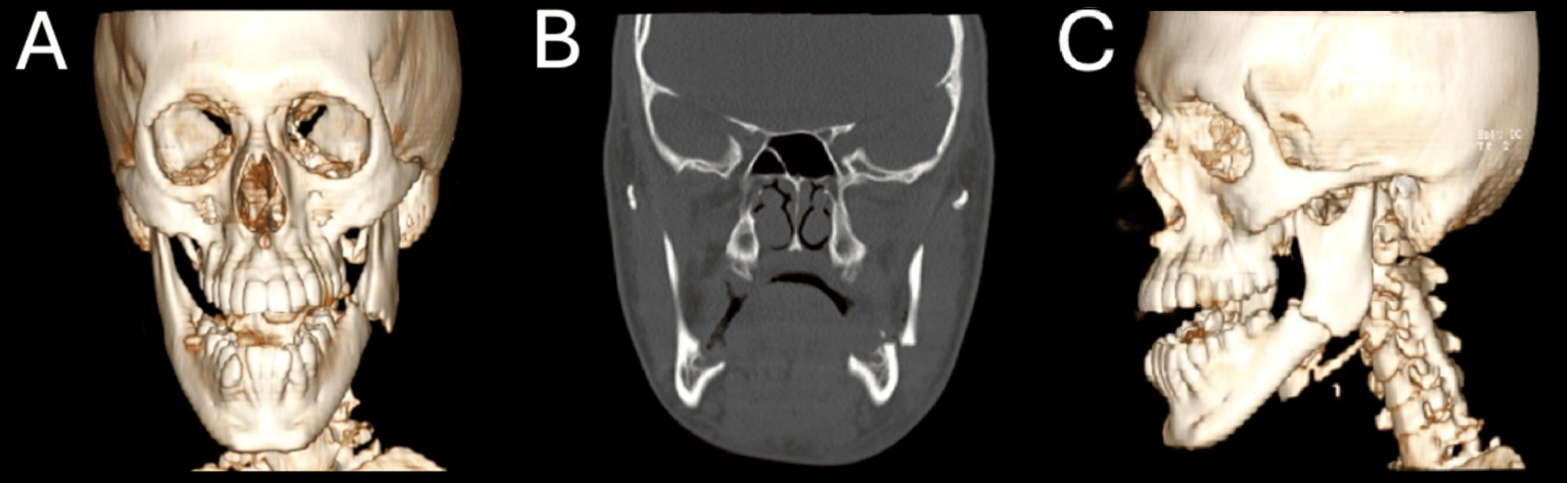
Acute left mandibular fractures on imaging with 3D reconstruction (A) CT maxillofacial with 3D reconstruction (coronal view) showing a mildly comminuted fracture of the angle of the left mandible. (B) CT maxillofacial (coronal view) demonstrating an acute, mildly comminuted fracture of the left mandibular angle, along with an old right mandibular fracture at the anterior body adjacent to the mental foramen. (C) CT maxillofacial with 3D reconstruction (lateral view). CT: computed tomography; 3D: three-dimensional
